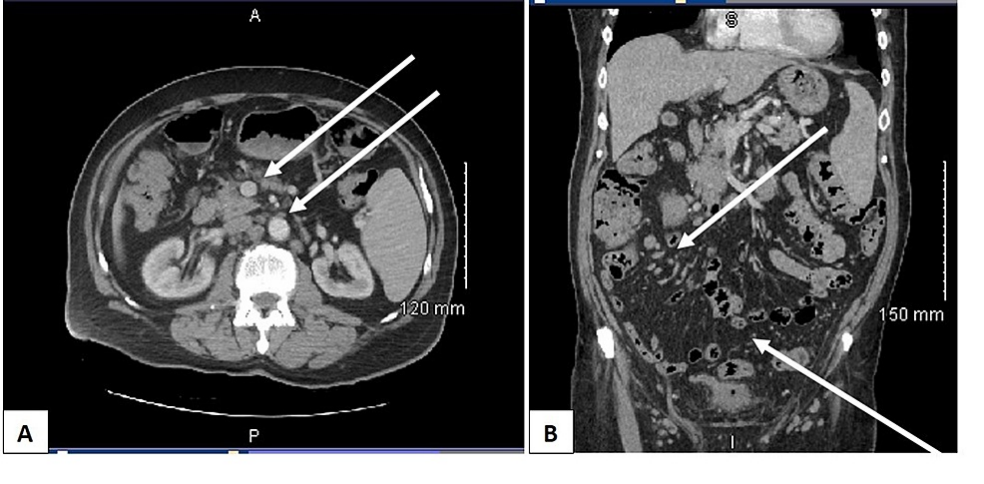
CT scan demonstrating the diffuse pelvic lymphadenopathy. The transverse (A) and coronal (B) planes with enlarged pelvic lymph nodes (arrows).
